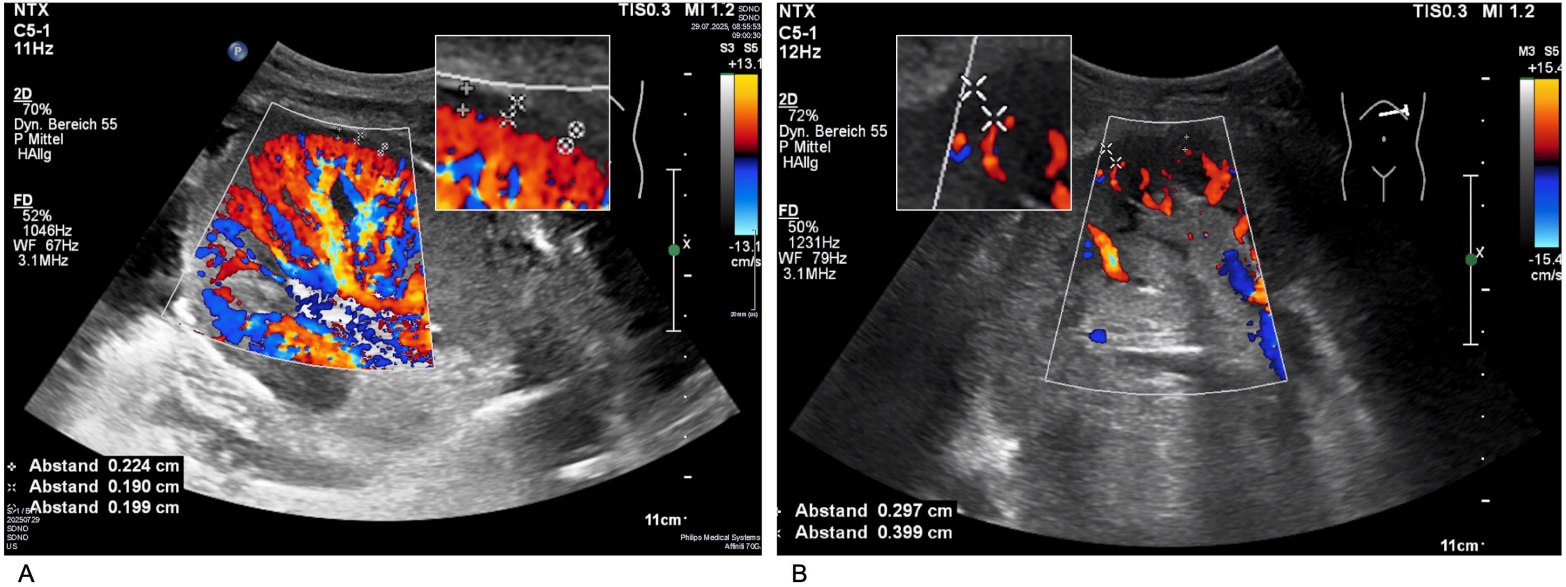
Ultrasound image of a well-perfused renal allograft with POV > 50% and PVD < 0.25 cm (A) versus image of a poorly perfused allograft with POV < 30% and PVD > 0.25 cm (B). POV, percentage of vascularization; PVD, periphery vessel distance.
